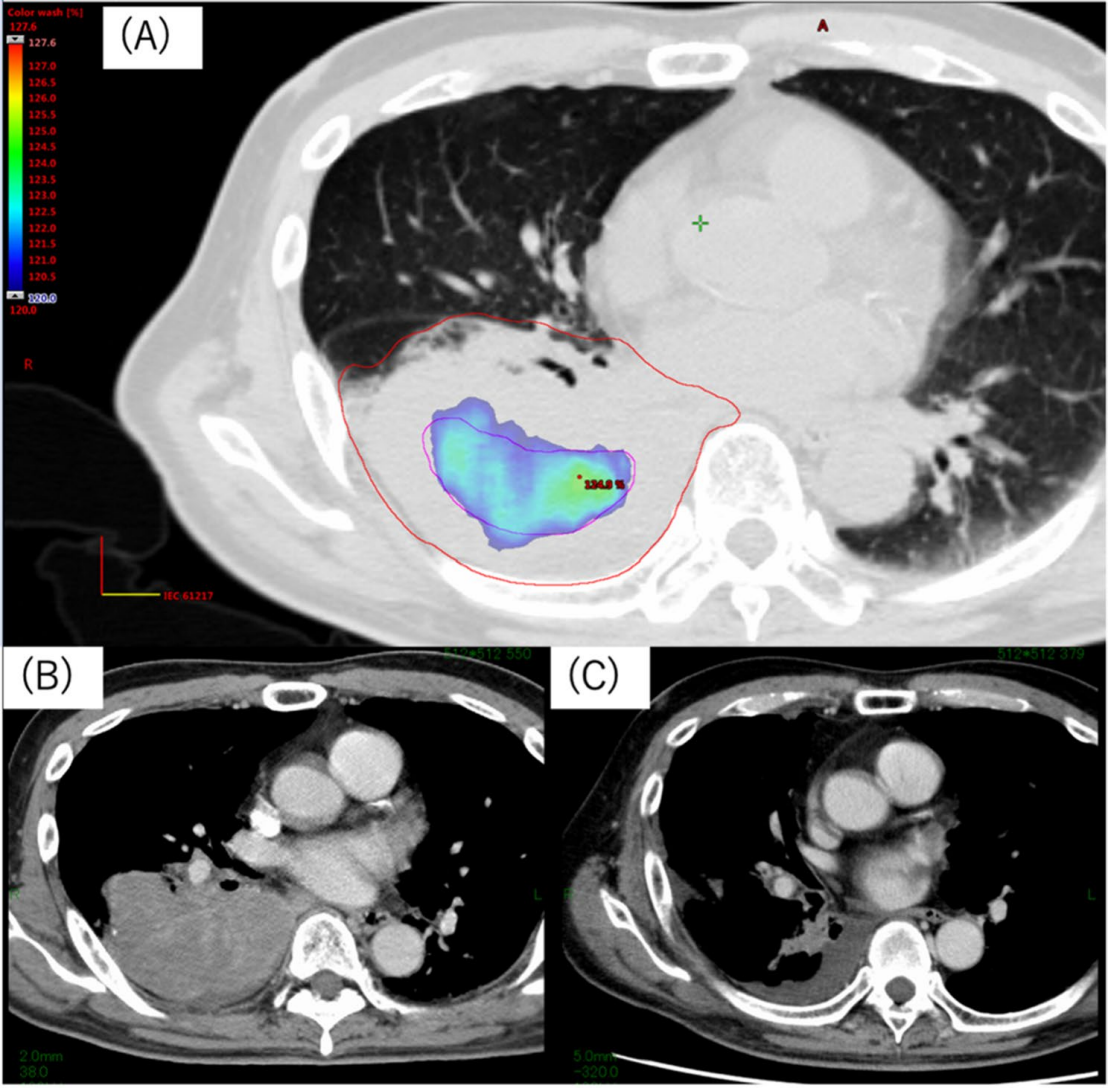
A patient receiving IIHDP VMAT. (a) An example of IIHDP VMAT. Dose-colour-wash area represents 120% of the prescription dose. (b) A CT image before the initiation of chemoradiotherapy, (c) A CT image acquired 5 months after the completion of chemoradiotherapy. CT, computed tomography; IIHDP VMAT, internal high-dose volumetric modulated arc therapy
